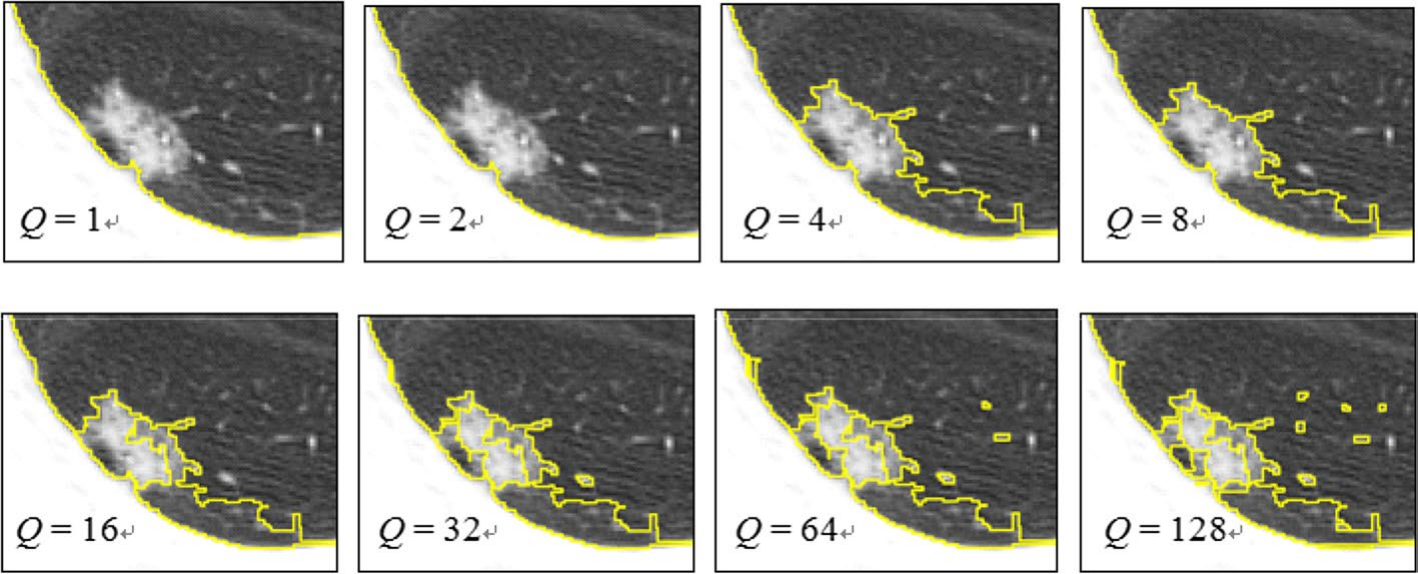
Segmentations of SRM on lung CT image, for various values of Q. Regions found are *yellow-bordered.*
